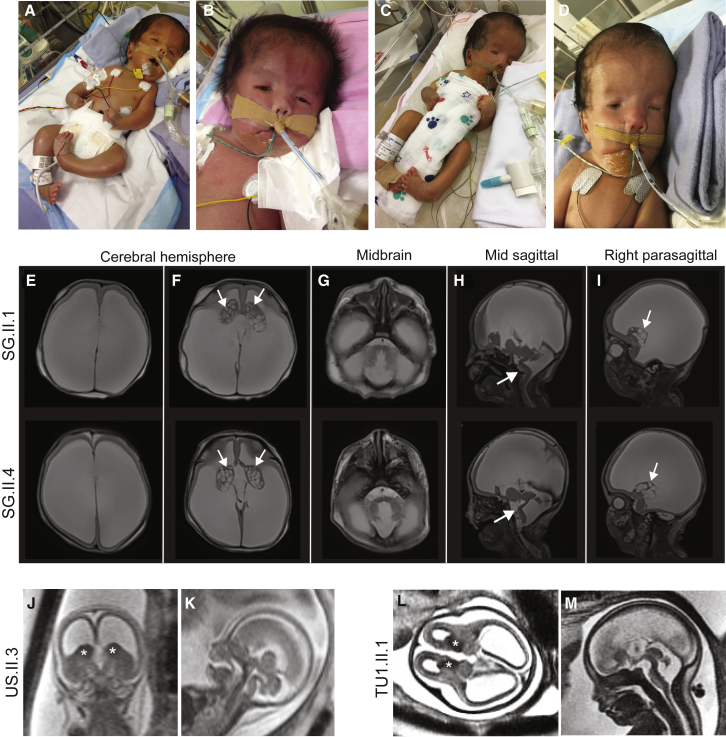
Pictures and Brain MRI Images of the SG.II.1 and SG.II.4 Babies and US.II.3 and TU1.II.1 Fetuses (A–D) Photographs of SG.II.1 (A and B) and SG.II.4 (C and D) babies showing their whole bodies (A and C) and a close up of their faces (B and D). (E–I) Brain MRI images of the elder brother SG.II.1 (top) and the younger brother SG.II.4 (bottom). Axial T2 weighted images showed severe ventriculomegaly, associated with severe thinning of the brain parenchyma (E, F). The brain parenchyma showed absence of normal gyral/sulcal pattern with smooth appearance in keeping with lissencephaly (E, F). Corpus callosum appeared to be absent (E, H). Note the prominent germinal matrix with germinolysis cysts (solid arrows) (F, I). The pons and cerebellum appeared hypoplastic with dilatation of the 4^th^ ventricle (G, H) and Z shaped appearance of the brainstem (solid arrows) (H). (J–M) Coronal (J), axial (L), and midsagittal (K, M) T2-weighed fetal prenatal MRI images of US.II.3 at 18.5 weeks of pregnancy (J and K) and TU1.II.1 at 28 weeks of pregnancy (L and M) demonstrating a similar imaging pattern including thin parenchyma (lissencephalic aspect), prominent germinal matrix marked by an asterisk, ventriculomegaly, and brain stem and vermian dysgenesis (kinked brain stem and elongated pons). In summary, we observe a similar brain malformation pattern both prenatally—US.II.3 in (J) and (K), TU1.II.1 in (L) and (M), AL.II.1 (see text), TU1.II.4 (see text), and TU2.II.2 (see text)—and postnatally (SG.II.1 [E–I top] and SG.II.4 [E–I bottom]).
